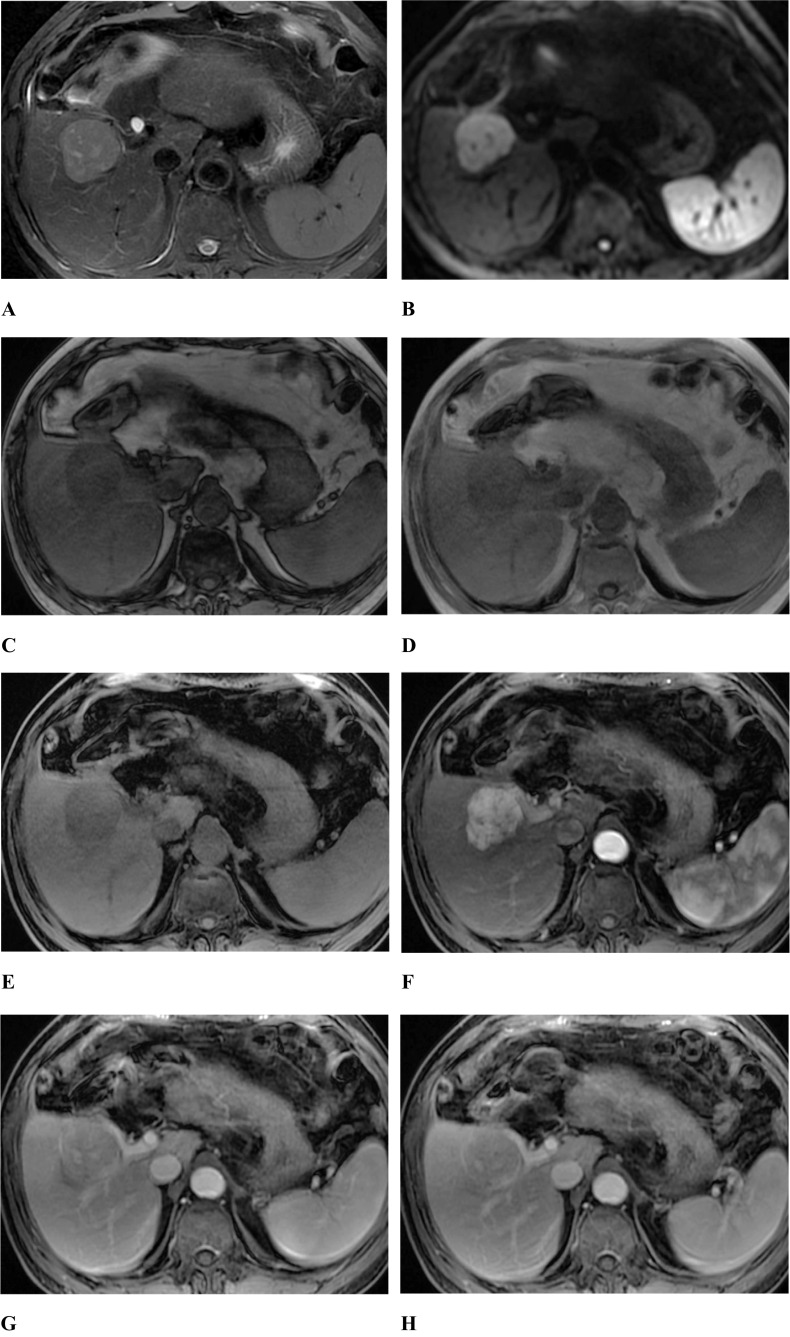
A 68-year-old male with NCPHCC. T2-weighted image with fat suppression showed a mass with slightly higher signal than in the hepatic parenchyma (A). Diffusion-weighted image (b = 800) showed a high signal mass (B). Tumor appeared as lower signal on out-phase image than on the in-phase image indicating steatosis, appeared as low signal on T1-weighted image with fat suppression before the enhancement (C). In the enhanced scan, the tumor showed fast-in and fast-out type, showing significantly enhancement in arterial phase (D), evidently decreased enhancement in portal vein phase (E) and false capsule with delayed enhancement in delayed phase (F)
